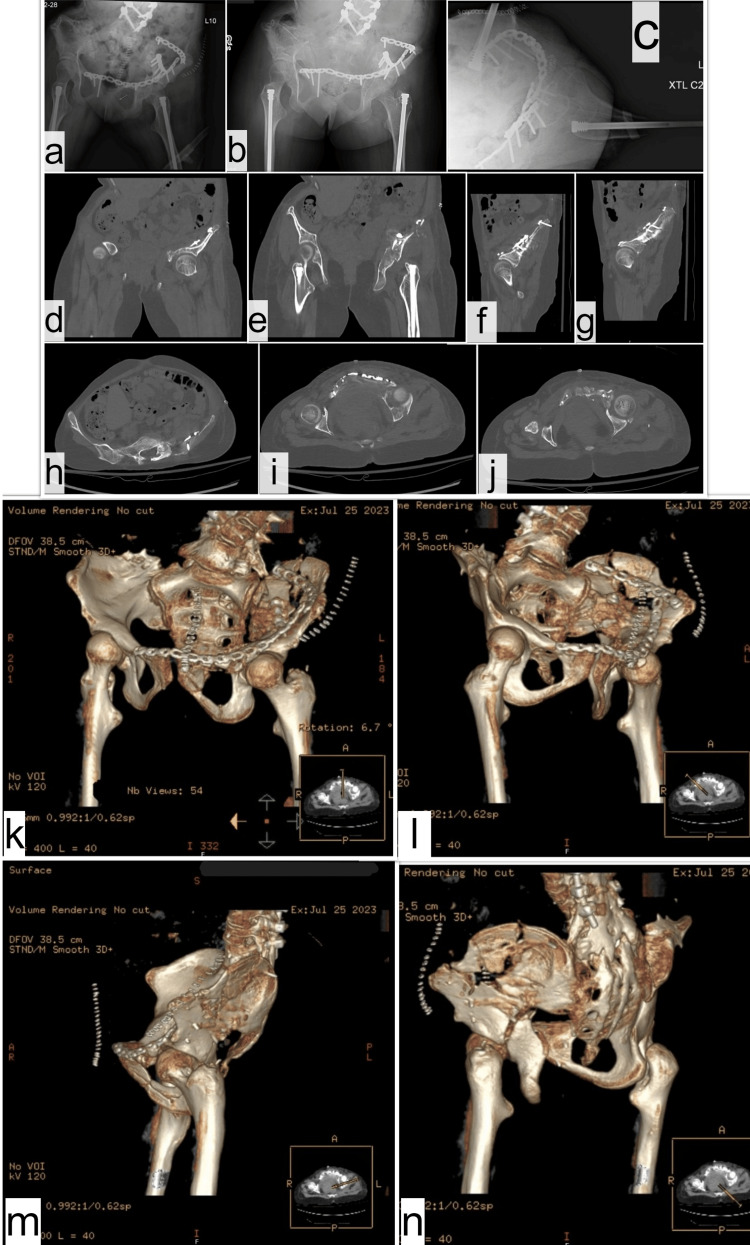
Postoperative imaging showing X-rays (A,B: AP pelvis), (C: lateral left hip), and CT scan (D,E: coronal), (F,G: sagittal), (H,I,J: axial), (K,L,M,N: 3D reconstruction) of reduced left-sided acetabulum fracture. AP: anterior-posterior
